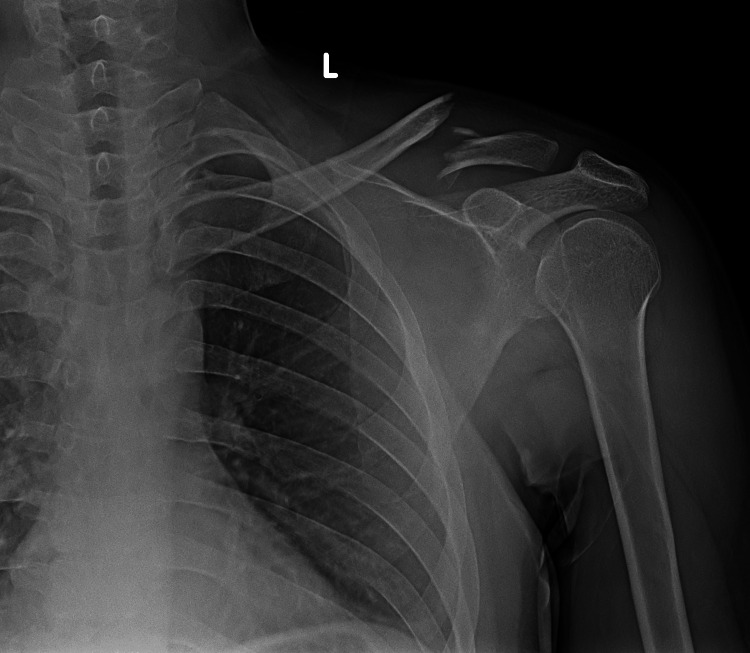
Initial X-ray scan showing a fractured clavicle
